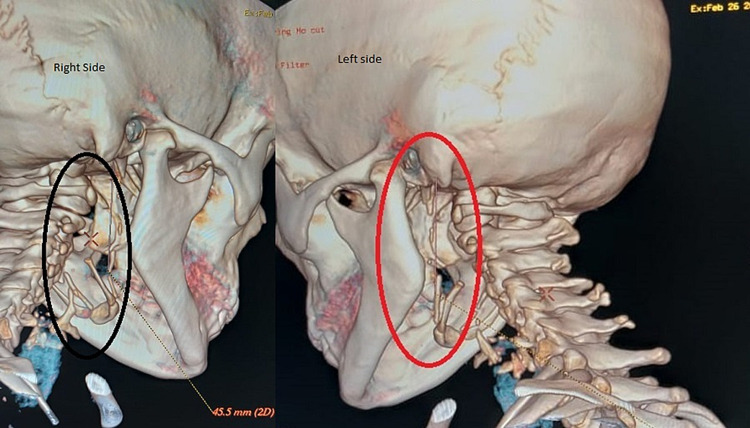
Three-dimensional CT reconstruction showing the elongated right and left elongated styloid process. CT: computed tomography
